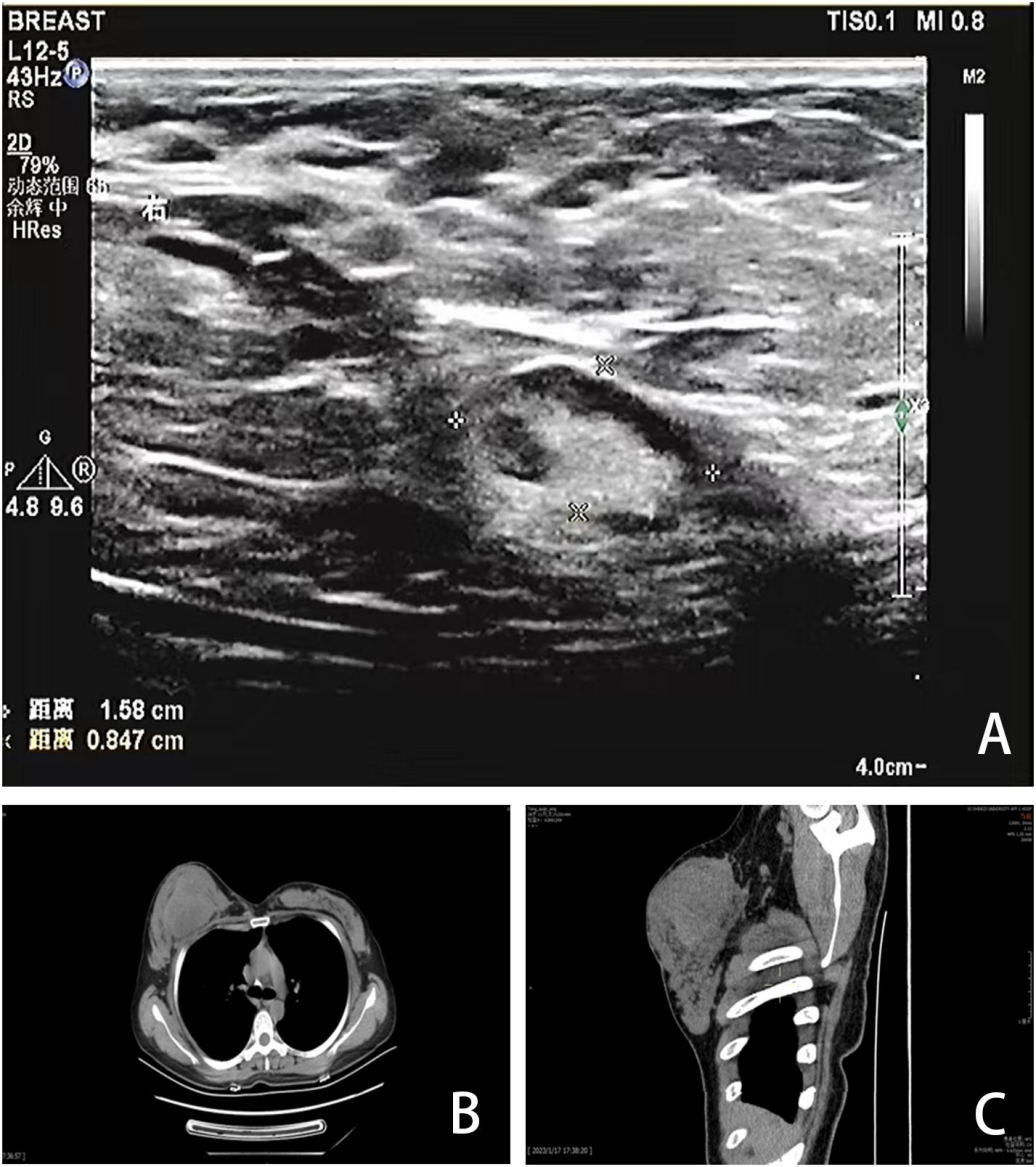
Imaging findings. (A) CDUS showed a heterogeneous mixed echo area of 9.7×6.0×10cm in the right breast. (B, C) CT showed a round mass in the right breast with patchy low-density patches and septations, with a diameter of 7.1×5.2cm.
